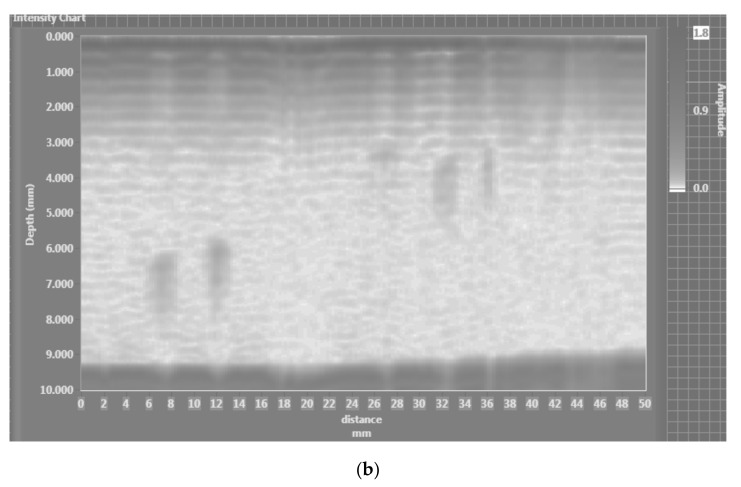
Ultrasonic measurement technique (a); data acquisition amplitude modulated scan; (b) ultrasonic imaging for the analysis of rail fracturing inside the subway line.
